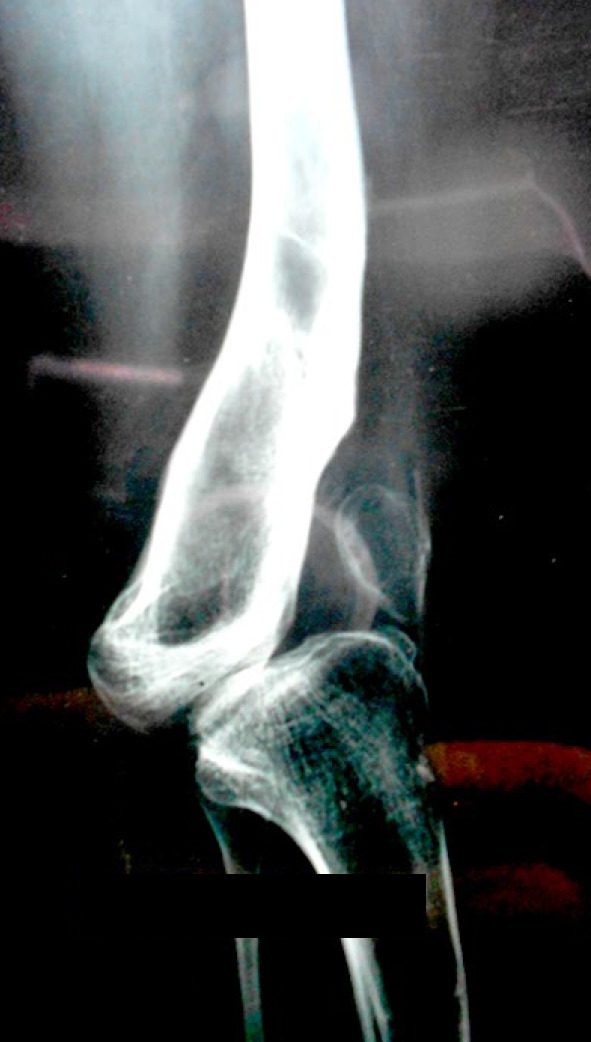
Radiographie prise 6 ans après une ostéotomie de flexion du fémur. Le recurvatum résiduel est compensé par la flexion fémorale, le tibia est subluxé en avant. La patiente se plaint de douleurs à la marche pouvant justifier la réalisation d’une arthrodèse du genou
